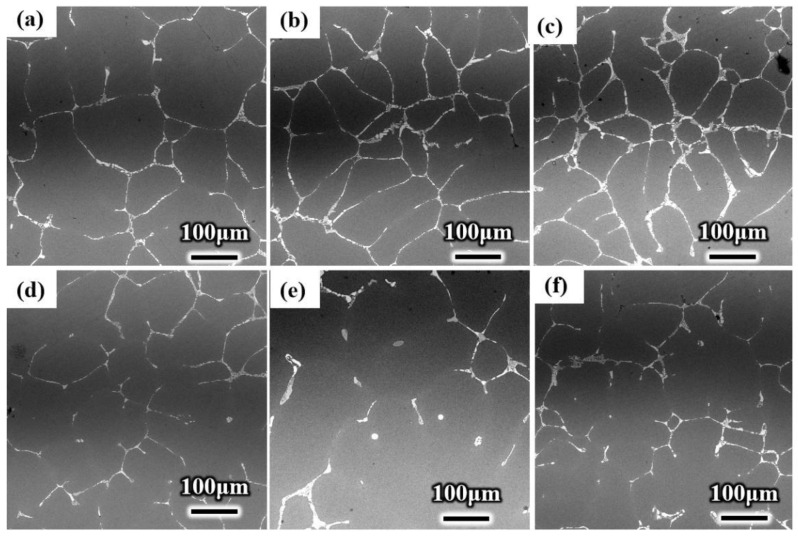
The eutectic phase distribution of the ultrasonic ingot and conventional ingot. (a) Edge of conventional ingot; (b) 1/2 radius of conventional ingot; (c) center of conventional ingot; (d) edge of ultrasonic ingot; (e) 1/2 radius of ultrasonic ingot; (f) center of ultrasonic ingot.
